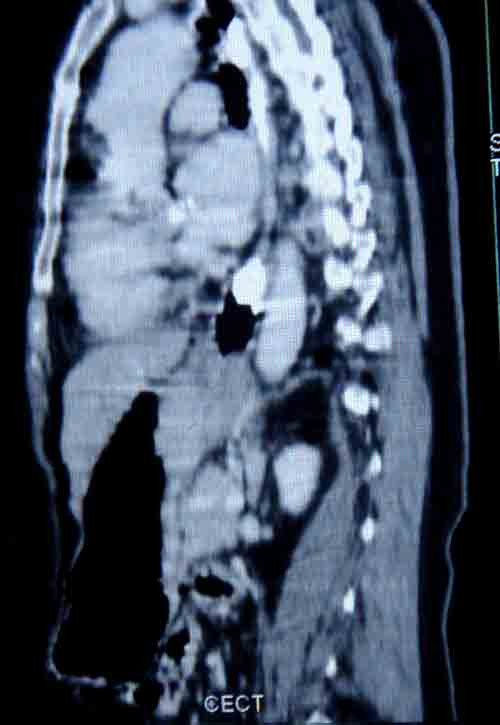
Computed tomography of thorax showing recurrence of growth in the lower part of the esophagus.
